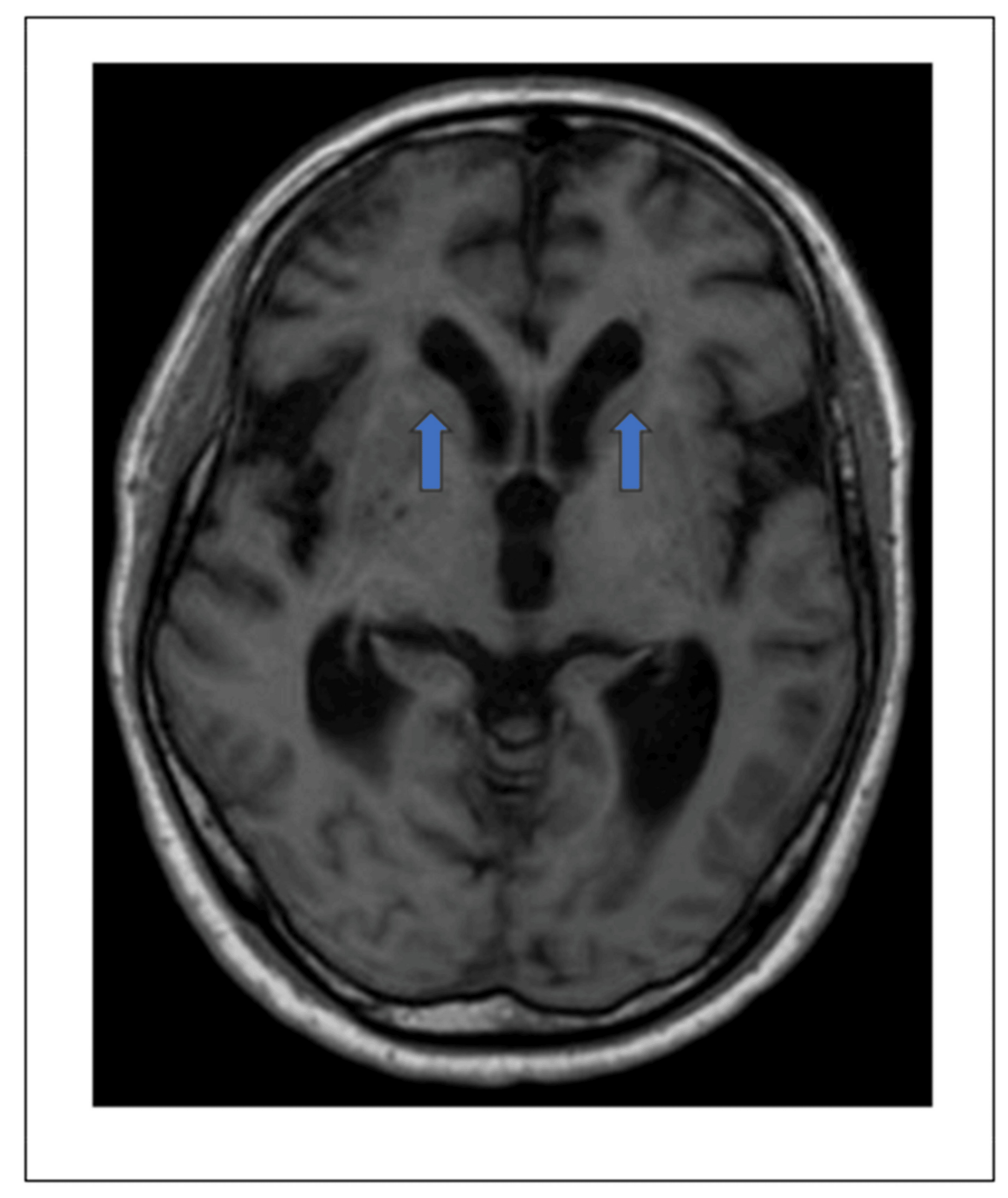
Cerebral magnetic resonance imaging
